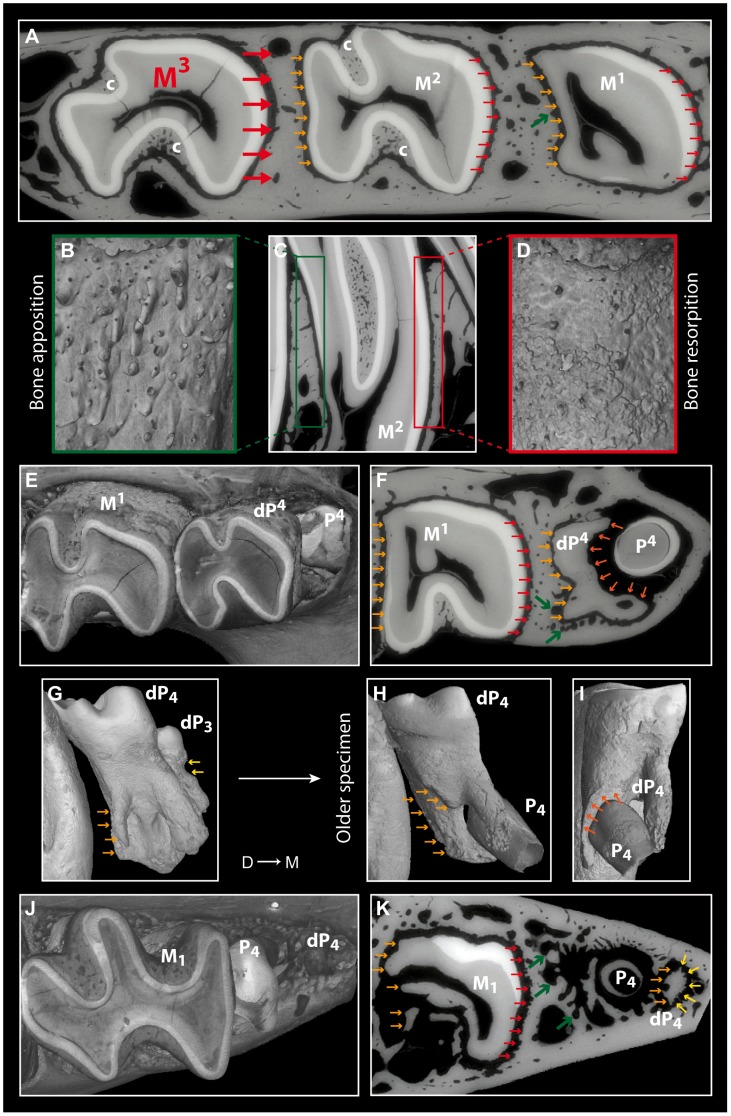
Bone and dental evidences of mesial drift in *Pectinator spekei*. A, F and K, X-ray synchrotron microtomographic virtual cross-section. C, X-ray synchrotron microtomographic virtual longitudinal section. B, D-E, G-J, X-ray synchrotron microtomographic 3D renderings. A-I, maxillary and upper cheek teeth (MNHN-CG1893-226 and 1895-461 for G); J-K, lower cheek teeth (MNHN-CG1893-226). Large red arrows display the orientation of compressive force; small red arrows indicate bone resorption by pointing the scalloped outline of alveolar wall; green arrows indicate bone apposition by pointing openings of vascular channels; small yellow to orange arrows indicate the different sites of dental resorption; c stands for cementum; D → M stands for distal to mesial direction.
